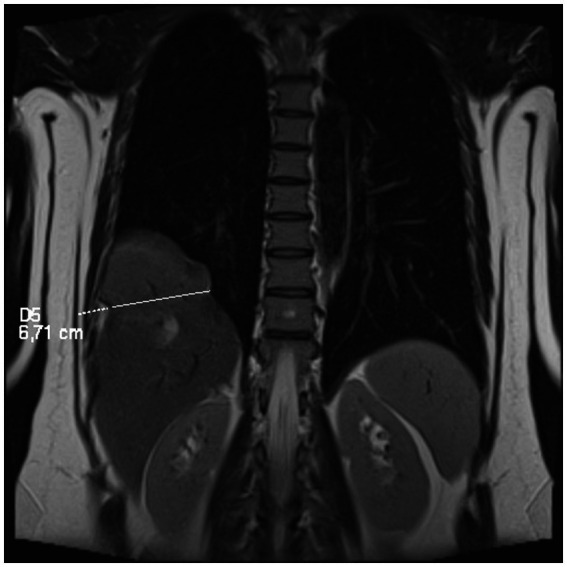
MRI image of liver herniation.
